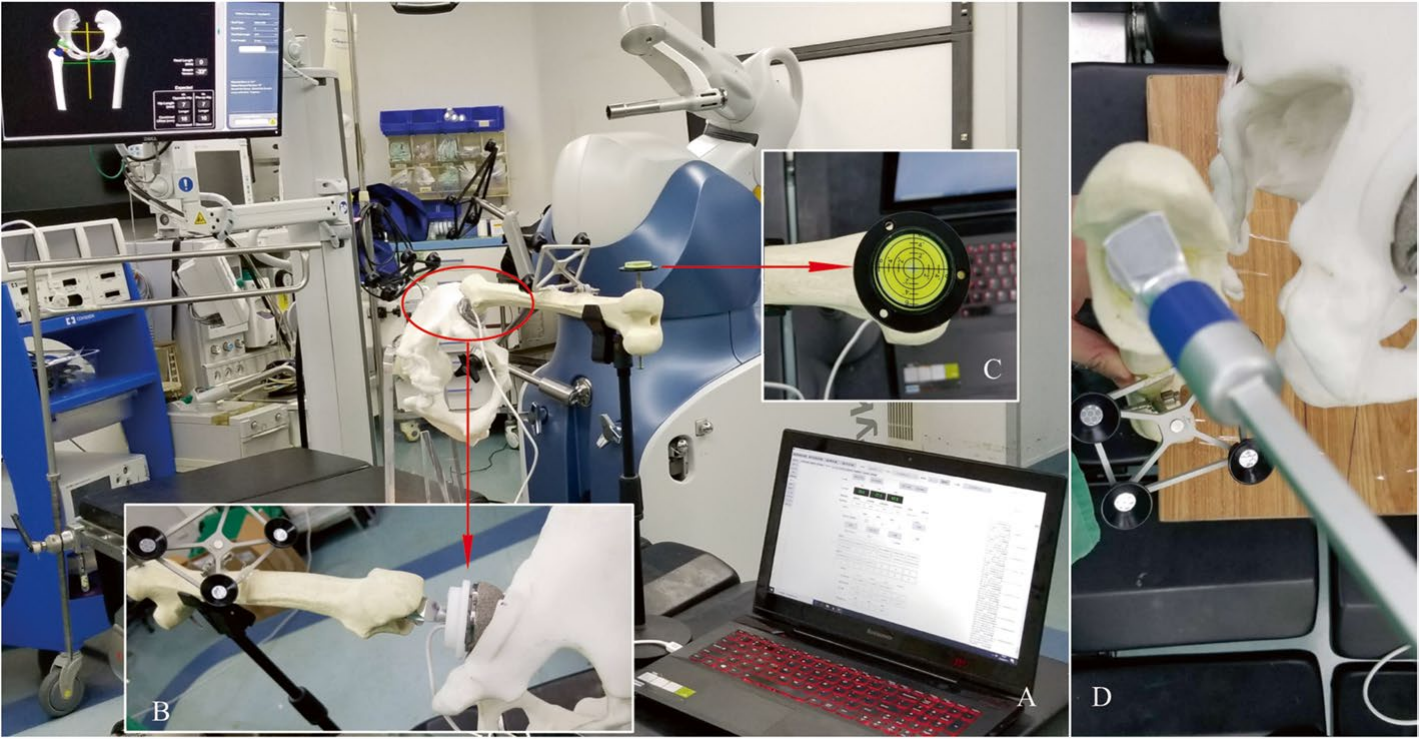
Experimental setting and measurement protocol of the robotic system and IMUHST. A The cup is inserted and assessed by the MAKO robotic system as the reference method, and the neutral position of the stem is recorded by the IMUHST to measure the vertical angle between this position and the gravity axis to calculate stem version (SV); B The stem neck is then rotated into a position perpendicular to the cup opening plane, guaranteed by a circular plastic mold. The angle between the initial neutral position and the current position is measured as the radiographic anteversion (RA), and the angle between the current stem neck axis and the horizontal plane is measured for calculation of radiographic inclination (RI); C The neutral position of the femur is guaranteed by a gradient put on a metal pin passing the trans-epicondyle axis. D The stem version can be adjusted for 5° increments between − 50° and 80°, and measured using the robotic system as a reference
